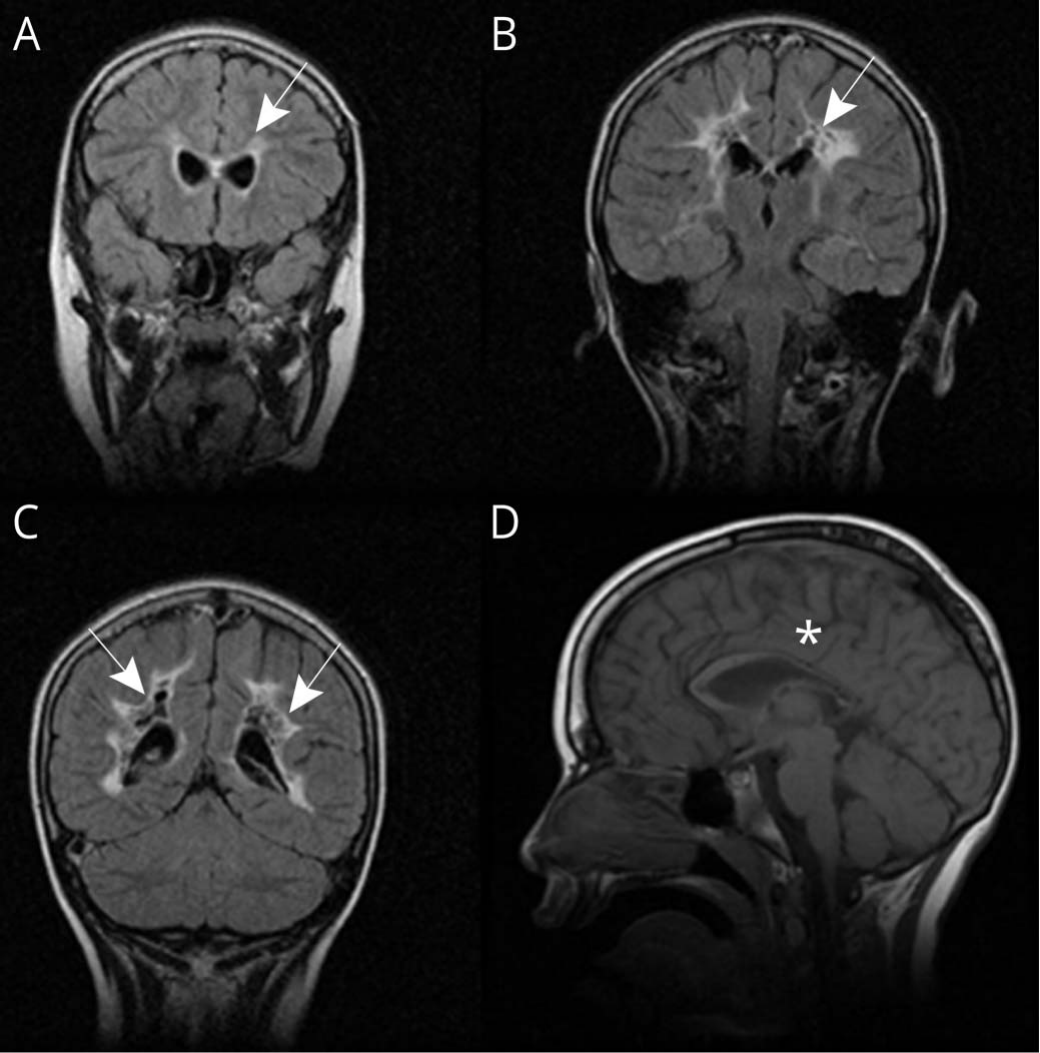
Neuroimaging MRI of the brain at age 8 ½ years with coronal T2-FLAIR FSE (TR/TE = 8,002/161.5 ms; A–C) and sagittal T1 (TR/TE = 440/18 ms; D) showing increased signal with multiple destructive cysts and localized atrophy in supratentorial white matter (white arrows; A–C) and involvement of the corpus callosum with atrophy and localized cysts with decreased signal (white asterisk; D). TE = echo time; TR = repetition time.
